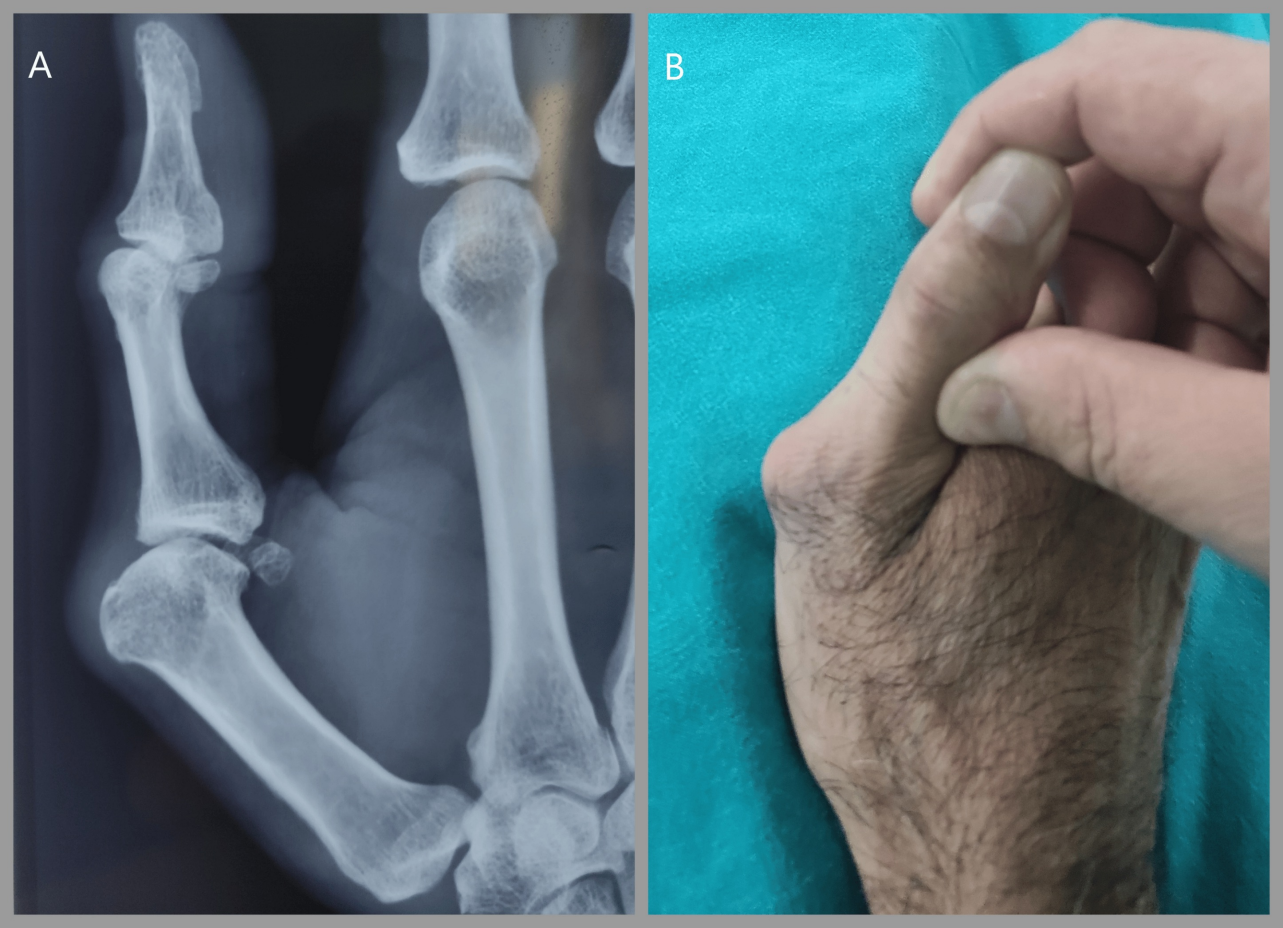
Preoperative images A: Plain lateral radiograph showing volar displacement of the proximal phalanx in relation to the metacarpal. B. A dorsoradial prominence was noted at the metacarpophalangeal joint
